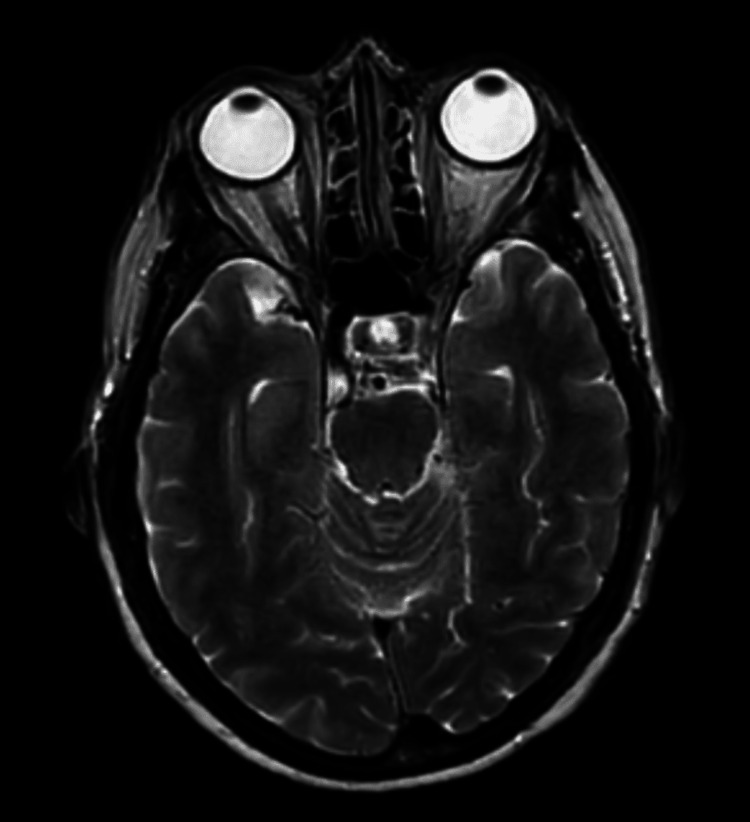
Normal axial T2 MRI head sequence from first presentation
